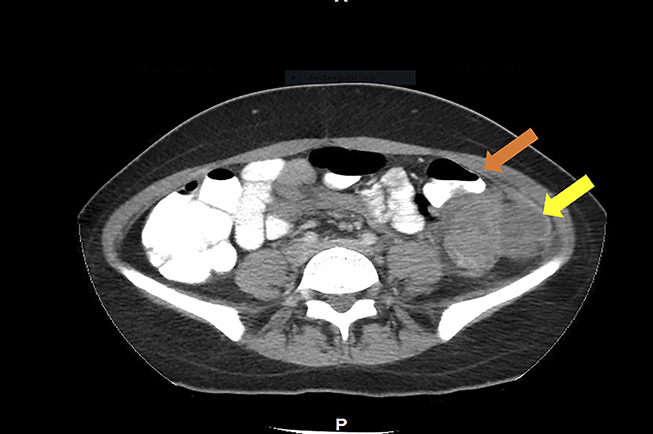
Abdominal and pelvic CT with oral and IV contrast (sagittal view) revealed severe mucosal thickening of a short segment of the descending colon measuring 5 cm in length (yellow arrow), causing severe lumen narrowing and partial obstruction of indeterminate etiology (orange arrow). CT, computed tomography; IV, intravenous.
